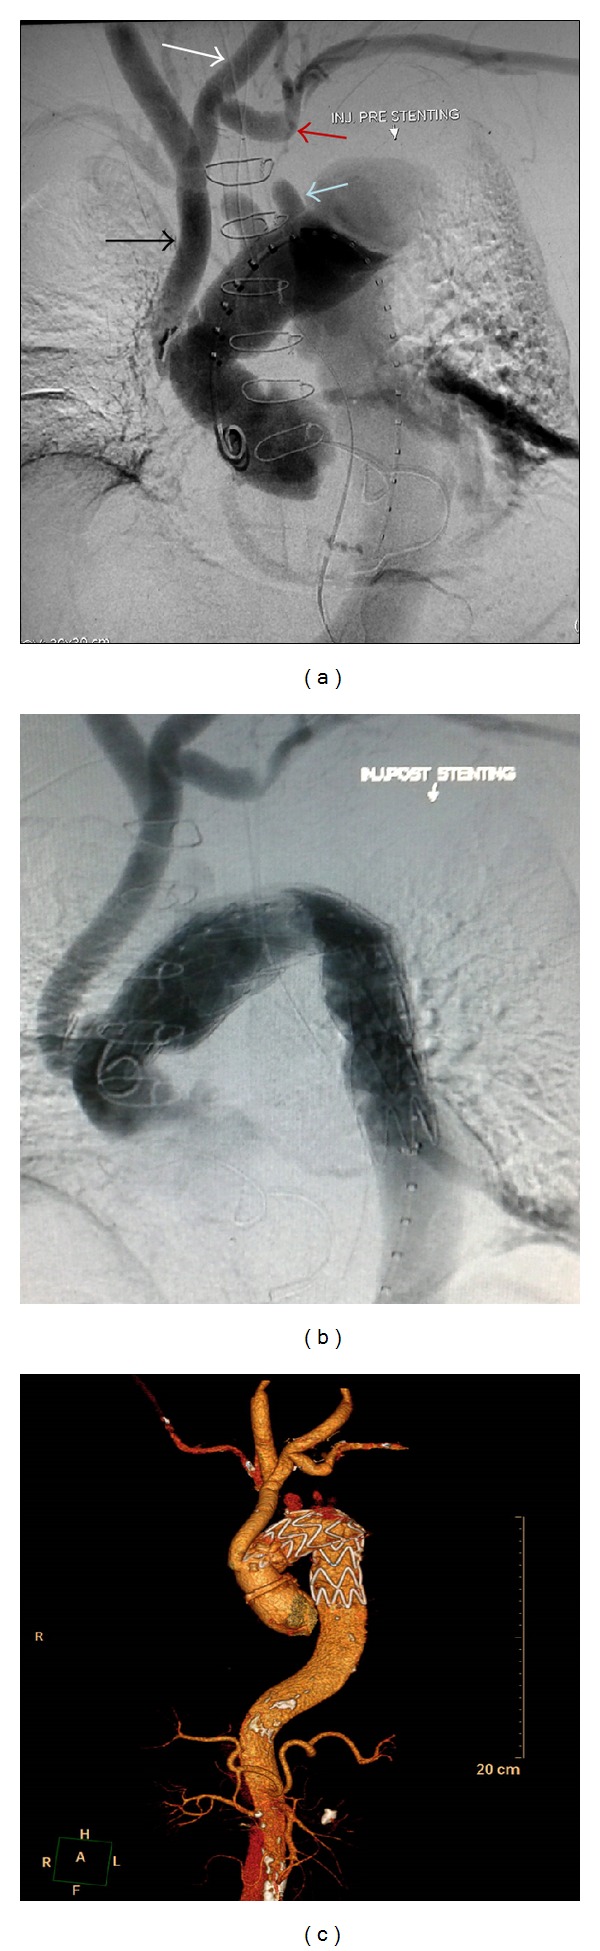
(a) Digital subtraction angiogram prior to stent graft deployment showing patent grafts taking inflow from previously placed ascending aortic graft with 10 mm graft to left common carotid artery (black arrow), piggybacking an 8 mm graft to left common carotid artery (white arrow), and another 8 mm graft to left subclavian artery (red arrow). The native stumps of the debranched arch vessels are also seen (blue arrow). (b) Check angiogram after stent graft deployment showing opened-up true lumen and nonopacification of false lumen. (c) CT angiogram, volume-rendered 3D image at 1 year follow-up showing patent bypass grafts, significantly remodelled aorta, and near-total thrombosis of false lumen.
